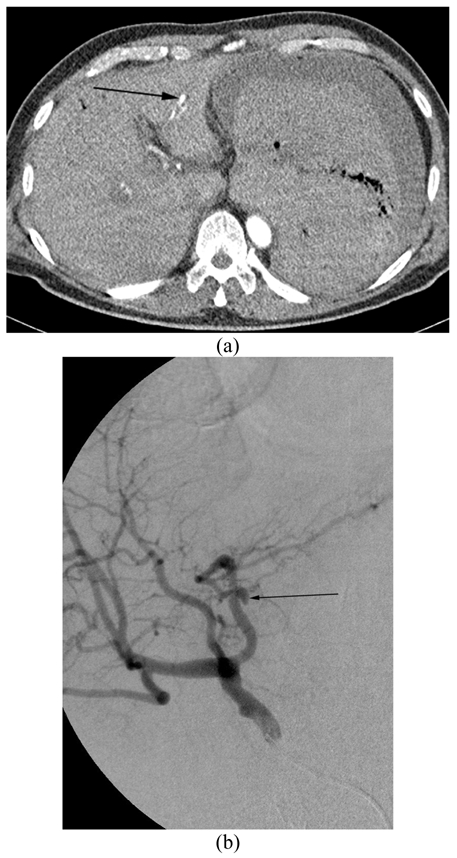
(a) CT angiogram shows 1 cm left hepatic artery pseudoaneurysm (arrow) within left lobe of liver. (b) CT angiogram immediately below the above lesion demonstrates active extravasation of contrast in the adjacent bile duct (arrow).
